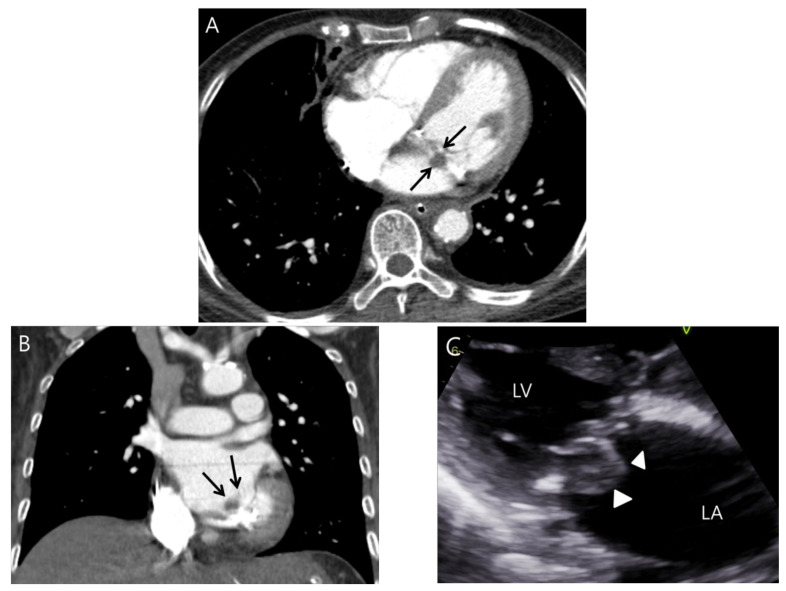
A case of nonelongated nodular mitral valve thickening observed on routine chest CT. A 69-year-old woman presented with fever. Axial (A) and coronal images (B) from routine chest CT show nonelongated nodular thickening (arrows) at the mitral valve. (C) Transthoracic echocardiography (TTE) demonstrates an echogenic vegetation with elongated appearance (arrowheads) on the mitral valve. LA and LV indicate left atrium and ventricle, respectively.
